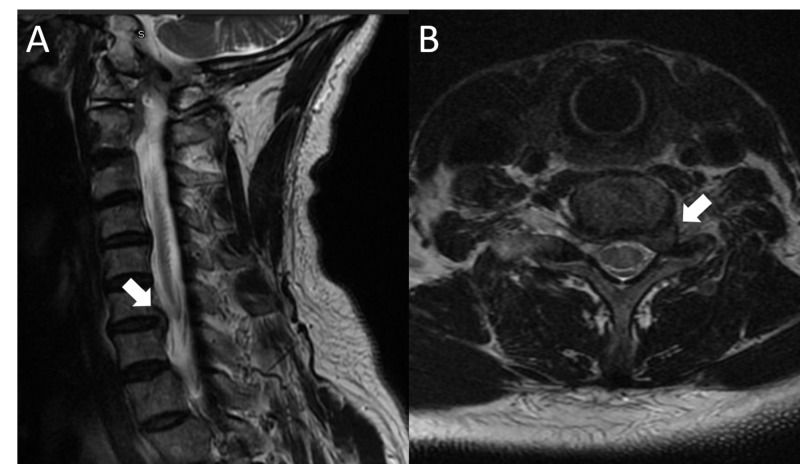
Preoperative cervical magnetic resonance imaging (MRI) (A) Sagittal T2 cervical MRI scan demonstrating a left C6-7 disc herniation. (B) Axial T2 cervical MRI at the C6-7 disc space, depicting a laterally protruding left disc herniation obstructing the neural foramen.
